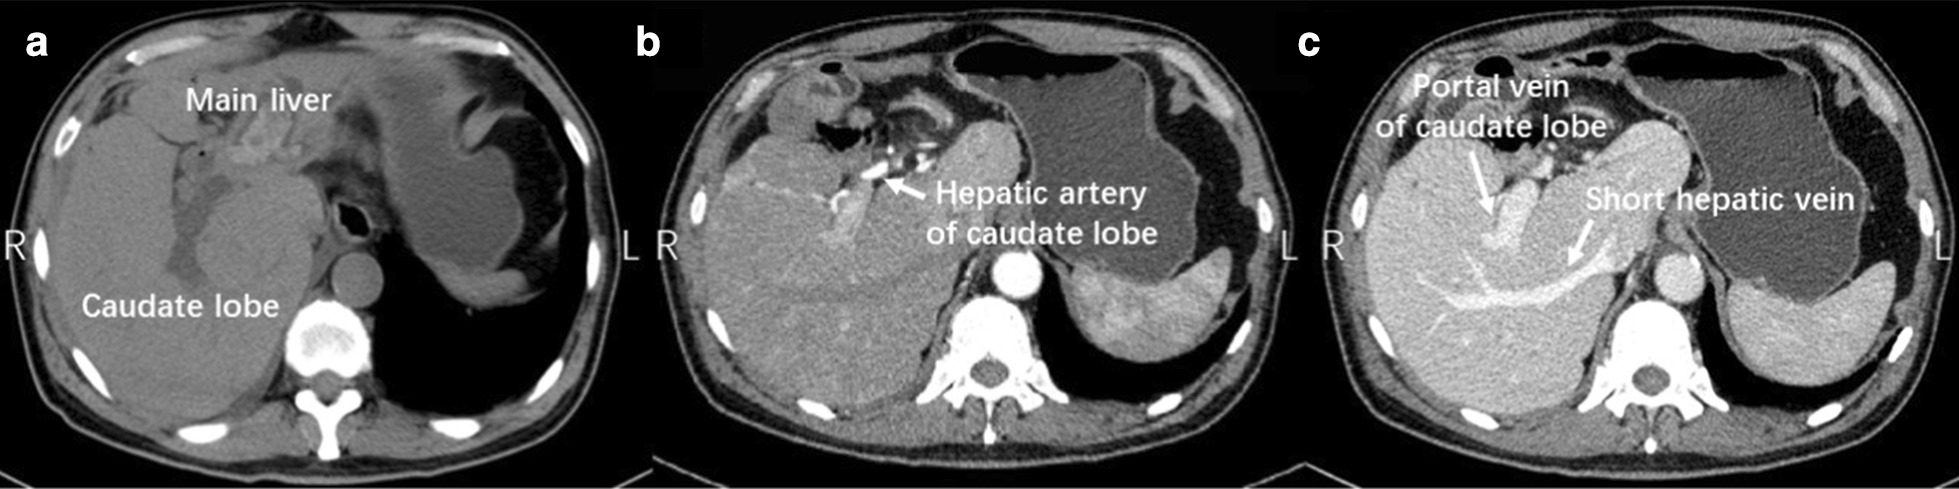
Imaging assessment of case 2. a CT image showed hypertrophic caudate lobe and hepatolithiasis in the main liver. b Contrast CT image showed hepatic artery of the caudate lobe. c Contrast CT image showed hepatic vein and portal vein of the caudate lobe
